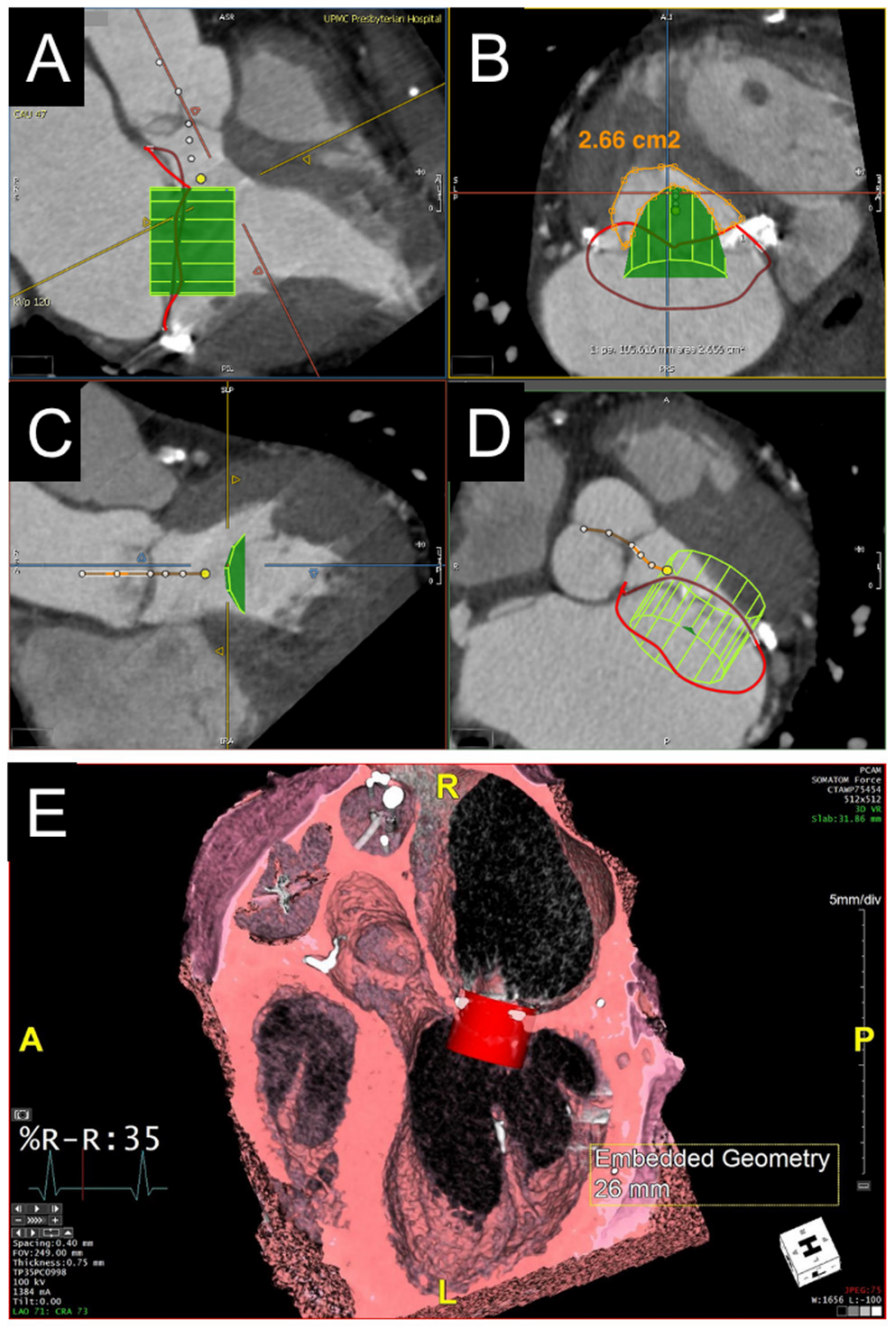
Evaluation of predicted neo-LVOT area by CT. (A–D) LVOT assessment for valve-in-MAC TMVR. Virtual implantation of a cylindrical transcatheter valve coaxial to the mitral annulus during a systolic phase (A) and delineation of the LVOT centerline yields a short-axis view of the predicted neo-LVOT area (B, *orange*), which measures 2.66 cm^2^ and predicts a low risk of LVOT obstruction with TMVR in this case. (E) Volume rendered image showing a capacious neo-LVOT area in a case under consideration for valve-in-ring TMVR.
